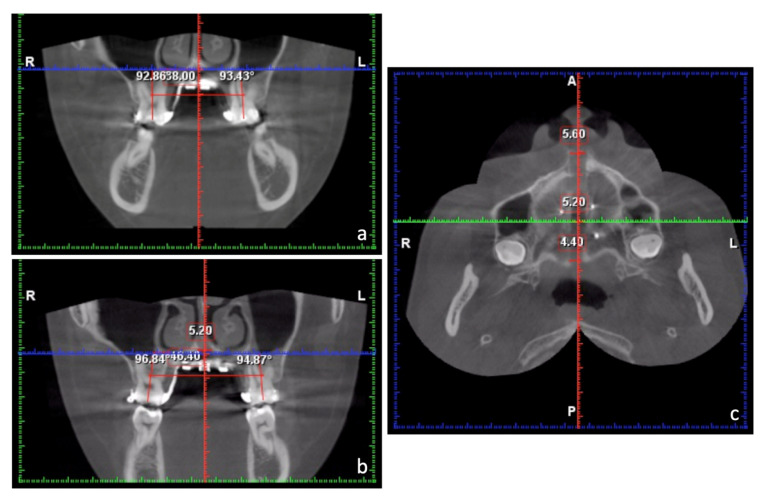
Measurements on the cone beam computerized tomography (CBCT). (a) Intermolar distance was defined as the distance between the trifurcations of right and left maxillary first molars. Molar angulation was measured between the axial plane and the long axis of the maxillary first molar. (b) Pre-expansion measurements: intermolar distance (38 mm) and molar angulation (92.86° on the right; 93.43° on the left). (c) Post-expansion measurements: intermolar distance (46.4 mm), intermolar expansion: (8.4 mm); molar angulation (94.84° on the right; 94.87° on the left), angulation changes (1.98° on the right; 0.44° on the left). In the axial plane, the amounts of skeletal expansion on palatal plane at anterior nasal spine (ANS), first molar and posterior nasal spine (PNS) were 5.6 mm, 5.2 mm and 4.4 mm, respectively. A fairly parallel expansion pattern was demonstrated in the antero-posterior (AP) direction (PNS/ANS: 78.6%).
